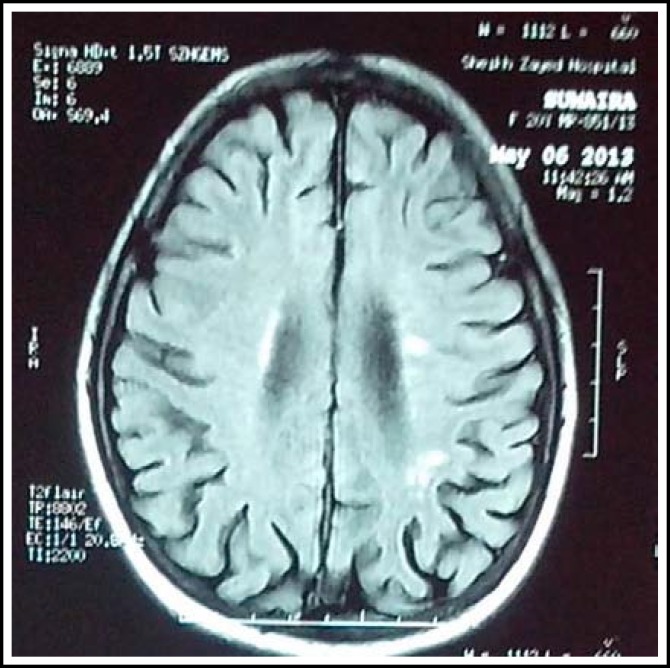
MRI showing demyelination in deep white matter (arrow).
